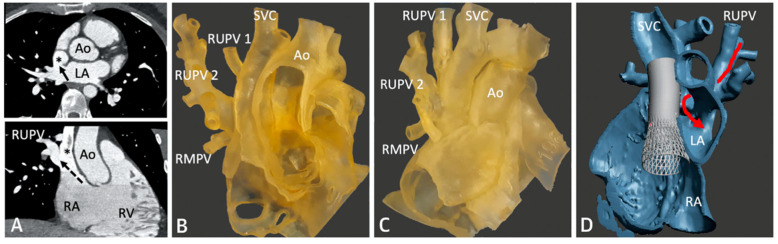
Series of images depicting pre-procedural planning with CT (A), three-dimensional (3D) printed models (B,C), and 3D virtual modeling (D). The defect is represented by an asterisk (*) in (A). After review with 3D imaging, each patient was either referred for covered stent placement (B, D) or surgical repair (C), if deemed inappropriate for percutaneous closure. Revised from ref [4].
